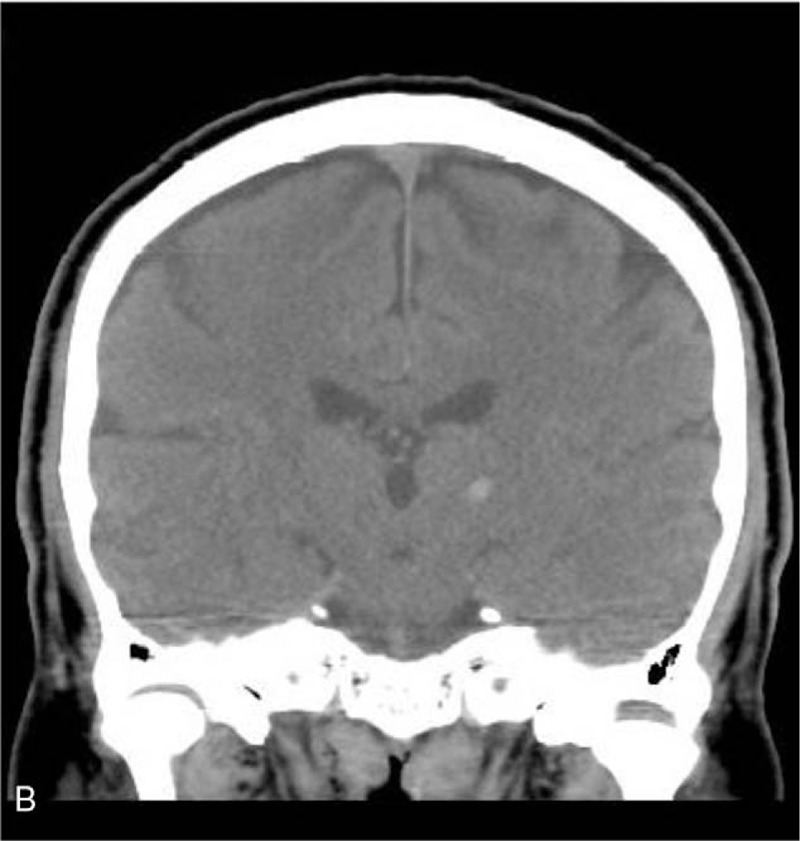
Computed tomography showing left thalamus hemorrhage (coronal view).
